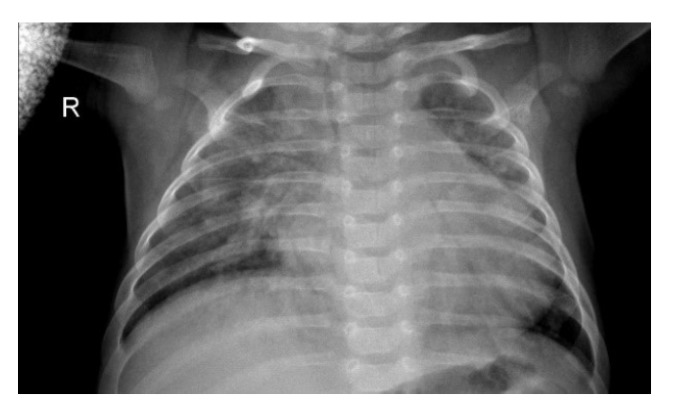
Chest X-ray (CXR) image depicting pneumonia (as can be seen by inflammatory condition of lung).
